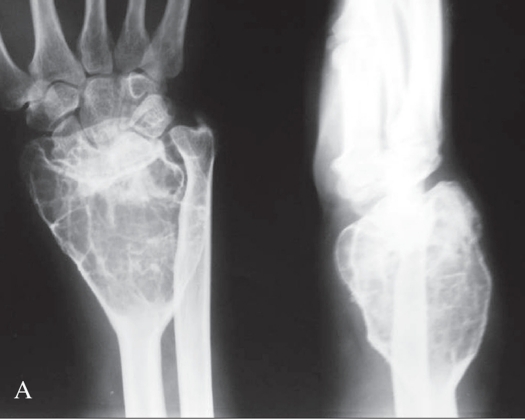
X-ray (A.P. and lateral) of distal radius shows campanacci grade 2 lesion
